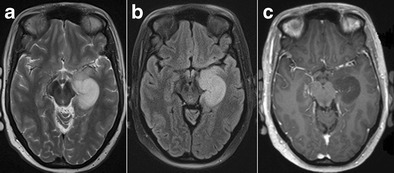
Low-grade glioma. 1.5-T axial FLAIR (a), T2W (b) and contrast-enhanced T1W images (c) show a T2-hyperintense, T1-hypointense, non-contrast-enhancing infiltrative mass in the left hippocampus, corresponding to a pathologically proven glioma
